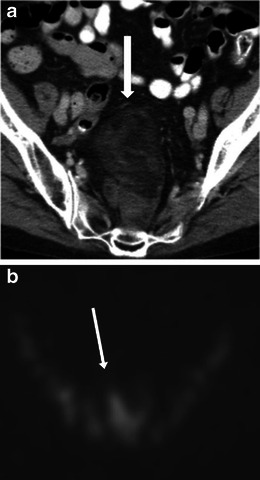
Imaging of myelolipoma. An 80-year-old woman with an incidental pre-sacral mass identified on CT (a). The mass (*arrow*) is heterogeneous with predominant fat attenuation and some solid enhancing components. The appearances are similar to a liposarcoma. Biopsy revealed mature adipose tissue and myeloid contents. A sulphur colloid scan (b) shows uptake in the pre-sacral region (*arrow*) confirming the diagnosis of extra-adrenal myelolipoma
